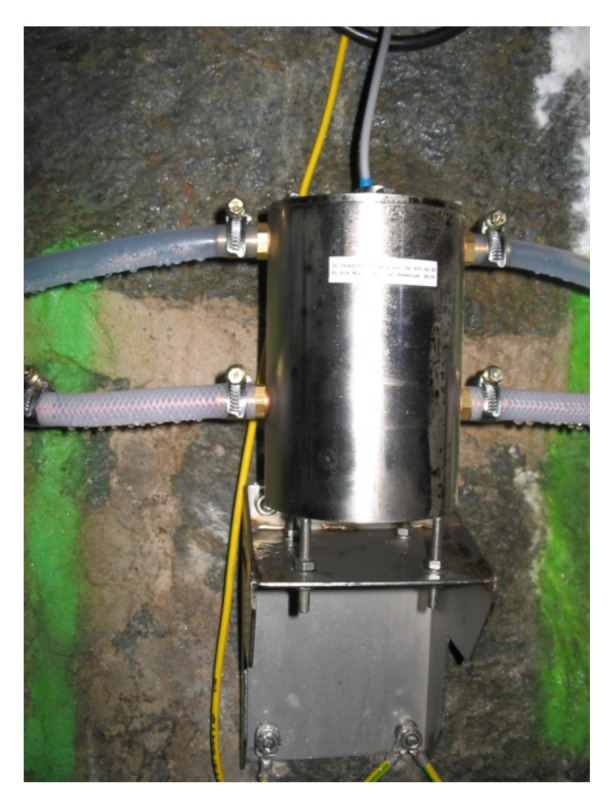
A UMP3 leveler unit with an ultrasonic sensor that was installed on one of the objects (appearance after a period of 15 years of use in harsh environmental conditions—high humidity).
